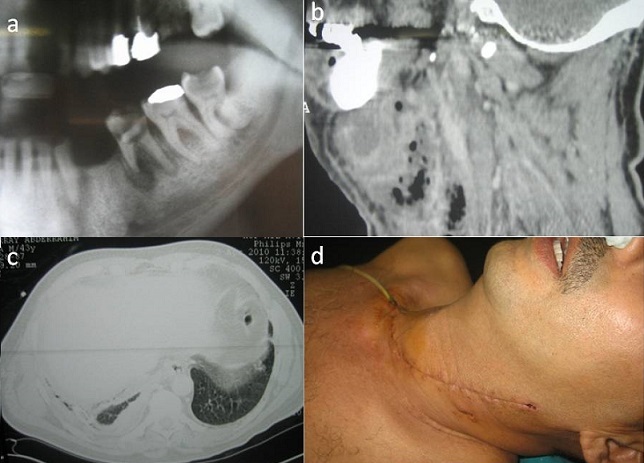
a) granulome inflammatoire de la 36 (dent causale) sur l'orthopantomogramme; b: infiltration cellulitique du tissu cellulo-graisseux cervical sur la tomodensitométrie (TDM) en reconstruction sagittale; c) comblement médiastinale (TDM en coupe axiale); d) drainage médiastinale
